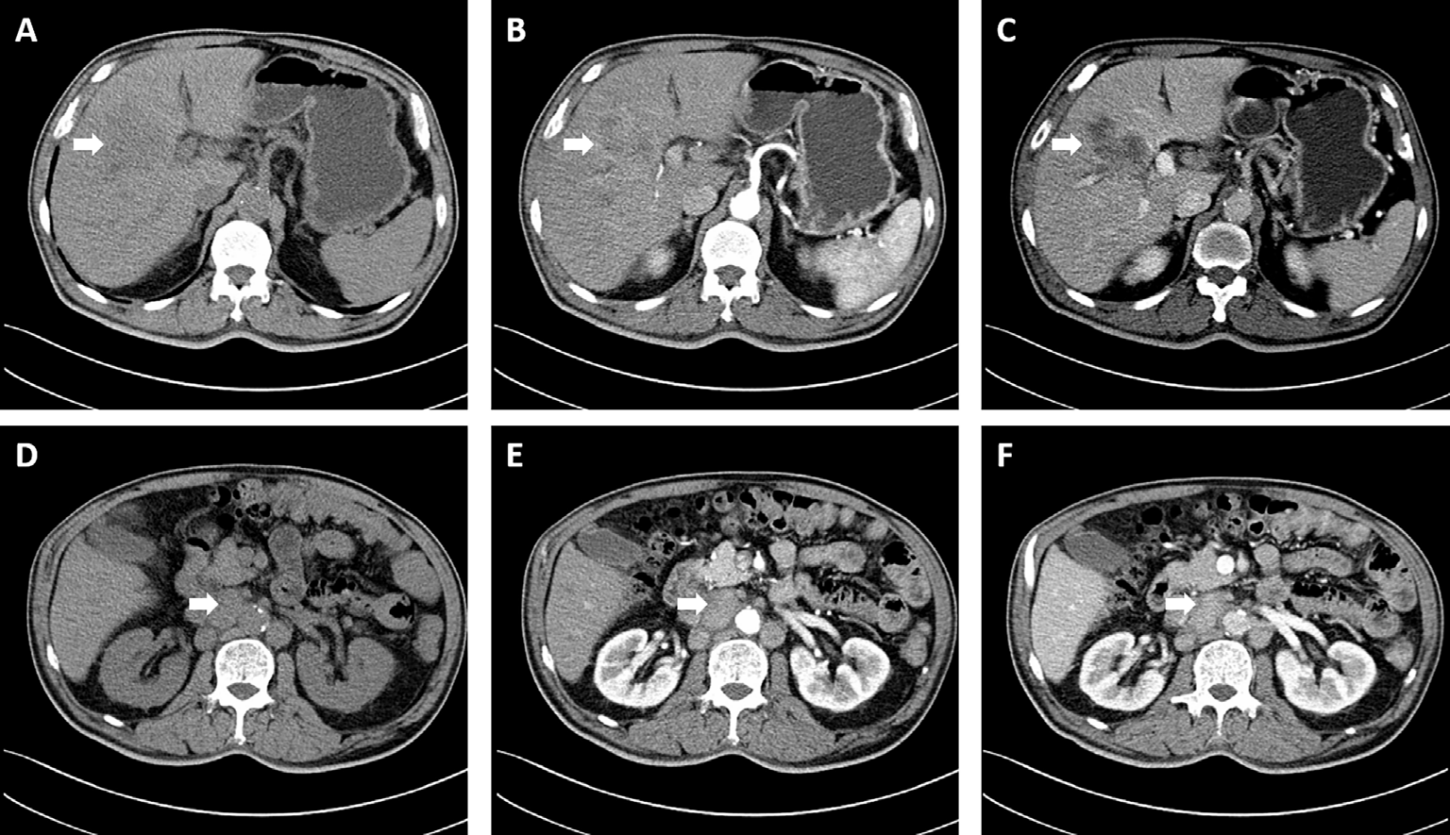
Computed tomography revealed an irregular morphology mass (49 mm * 39.6 mm) in the liver; the hepatic portal and retroperitoneal lymph nodes were enlarged. (A, D) Plain scan; (B, E) Arterial phase; (C, F) Portal venous phase. The white arrowheads direct the lesion or enlarged lymph nodes.
